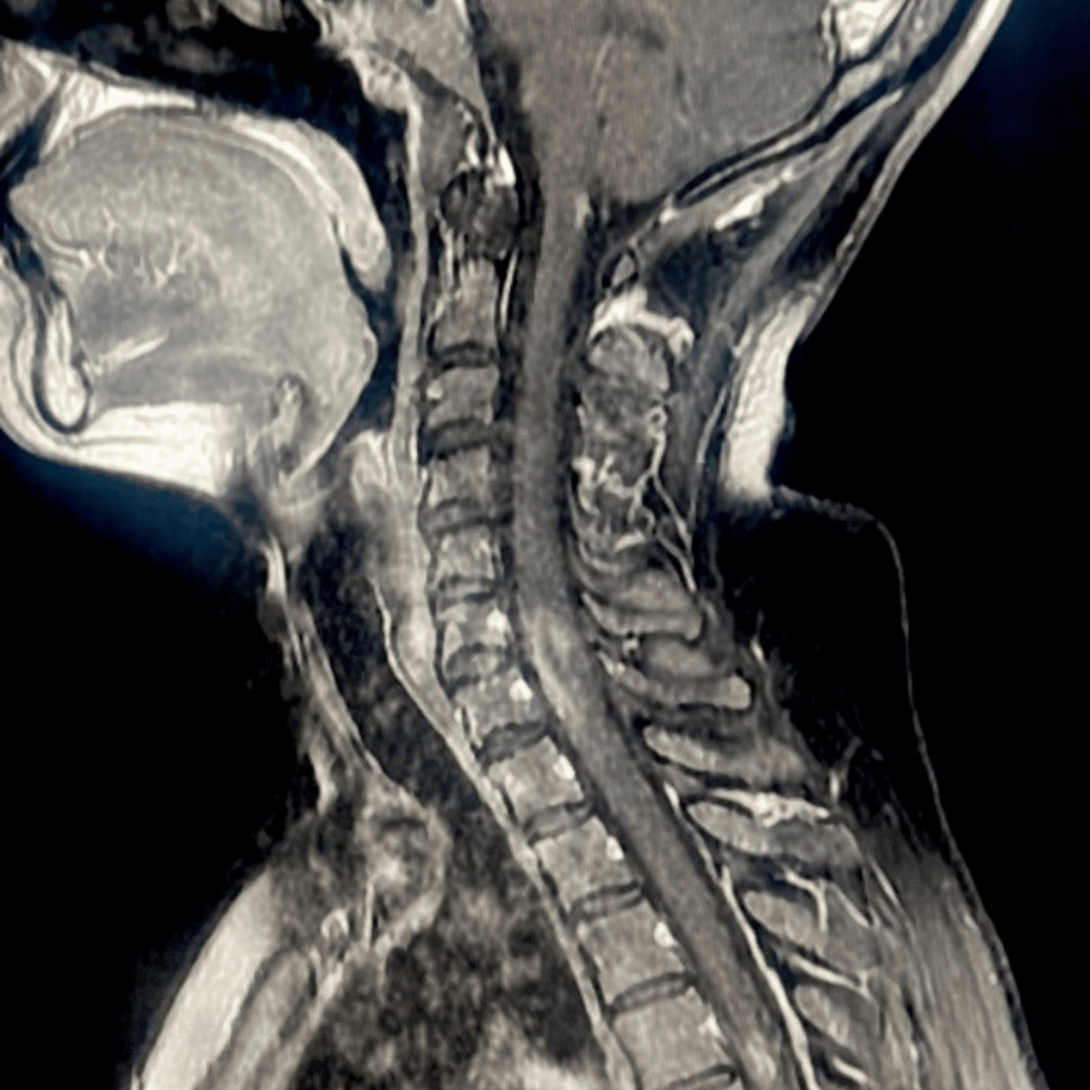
MRI of the spinal cord There is a focal right posterior lesion in the corticomedullary junction, involving short-segment lesions at the cervical and upper dorsal spinal cord levels.
